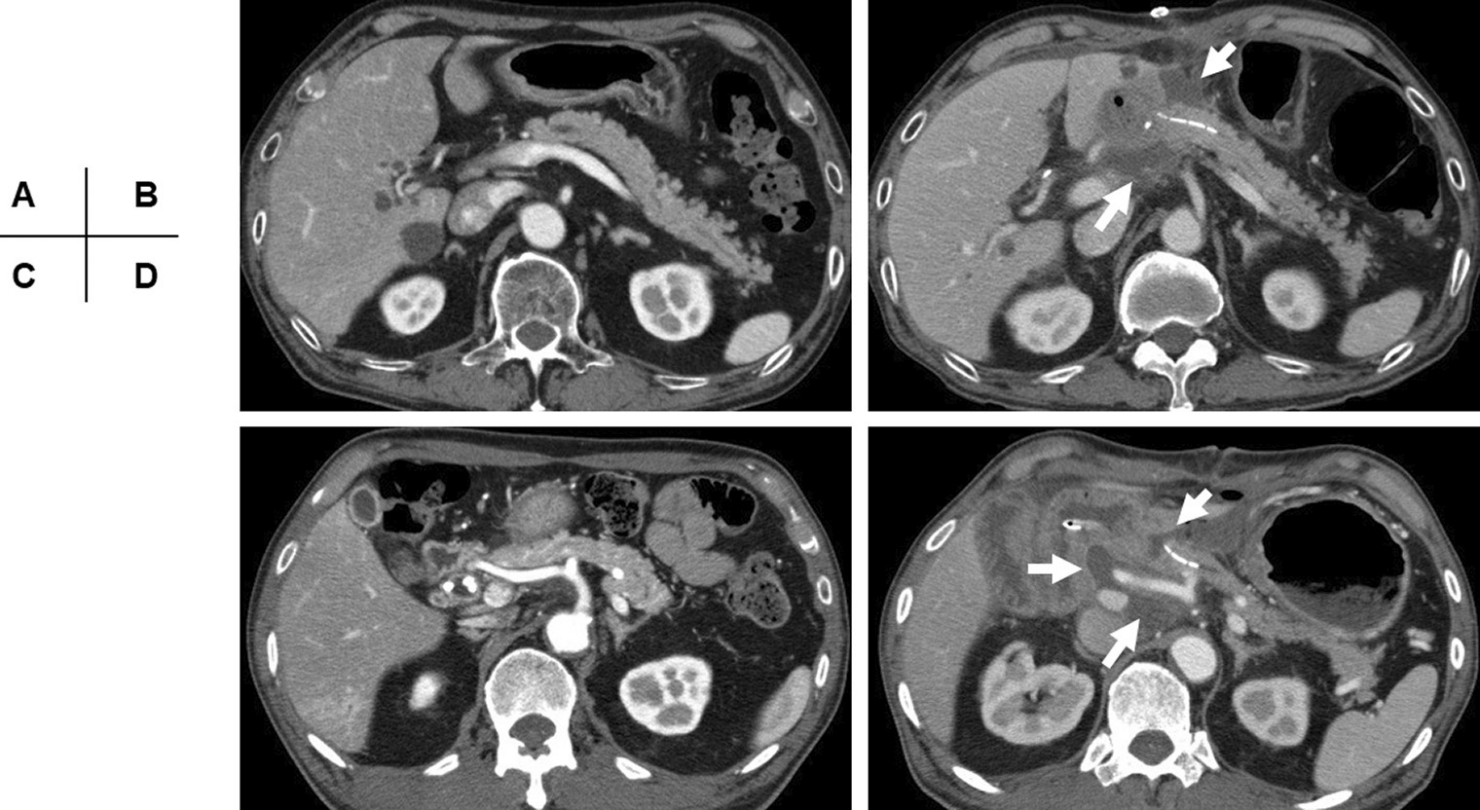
Representative CT findings of POPF without postoperative acute pancreatitis. Preoperative (A,C) and postoperative (B,D) CT findings in each patient. (B,D) postoperative CT evaluations demonstrating fluid collection solely around the pancreatojejunostomy (arrows) that is not accompanied by morphological changes in the remnant pancreas. Peripancreatic inflammatory changes are also not observed. CT, computed tomography; POPF, postoperative pancreatic fistula
